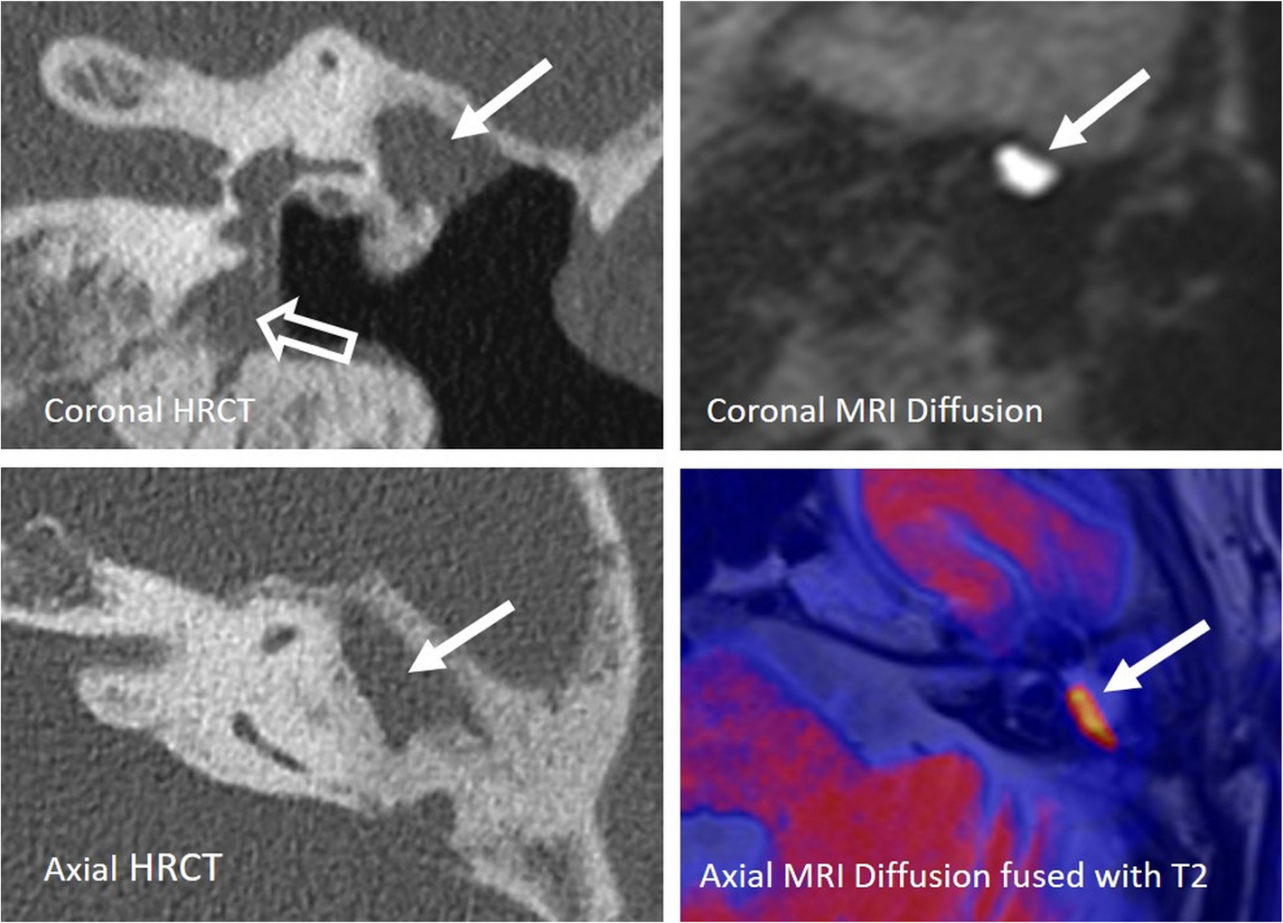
A 80-year-old male patient, with severe SNHL enrolled for cochlear implant surgery. The patient had chronic middle ear infections and cholesteatoma surgery with tympanoplasty type IV several years ago. HRCT coronal and axial images (left images) show opacified antrum (arrow) and opacified hypotympanon (open arrow). A recurrent cholesteatoma cannot be ruled out. Corresponding MRI diffusion coronal image and color-coded axial image fused with T2 (right images) show a high signal intensity in the opacified antrum, typical for cholesteatoma (arrow). The diagnosis was surgically verified, and the patient successfully received a CI one month after revision surgery
